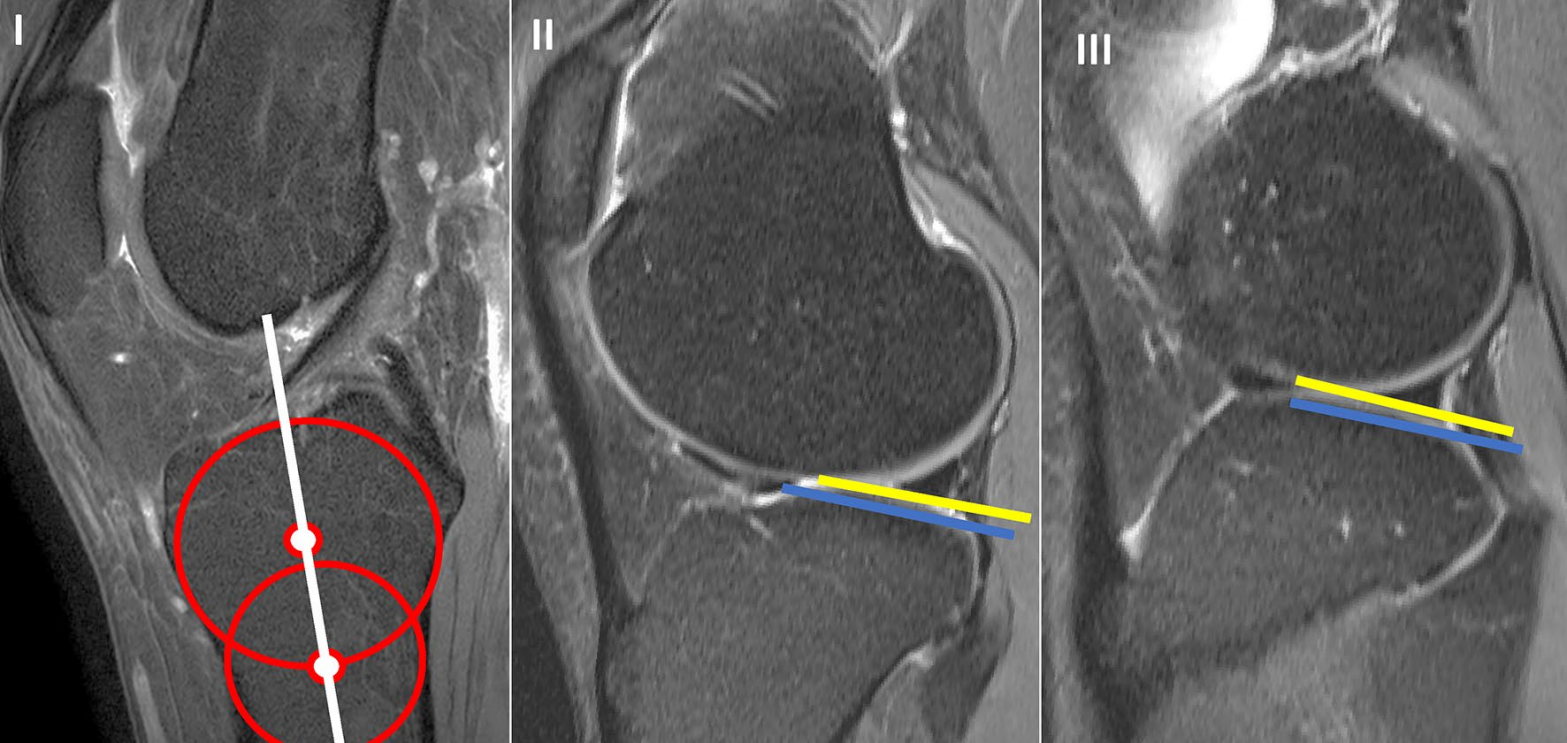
Measurement of tibial slope in the PLC and PLL segment according to Hudek et al. in a 31-year-old healthy woman. It shows the determination of the tibial shaft axis. The white line represents the tibial shaft axis and is defined by the centers of the two red circles. The proximal red circle is bounded by the anterior and posterior corticalis and the tibial plateau. The distal red circle is bounded by the anterior and posterior corticalis and the center of the circle lies on the circumference of the proximal circle; II sagittal MRI in the center of the PLC segment, the yellow line is the tangent representing the cartilaginous slope and the blue tangent represents the bony slope; III sagittal MRI in the center of the PLL, again the yellow line represents the tangent presenting the cartilaginous slope and the blue one the bony slope
